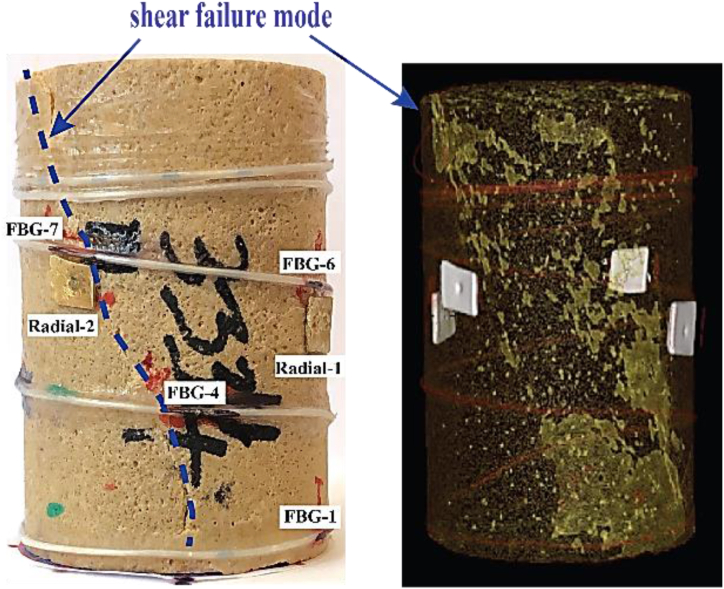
Post-test photo (left) and 3D rendering of the main open structures and segmentation of X-ray CT images of the Sav-3334.
